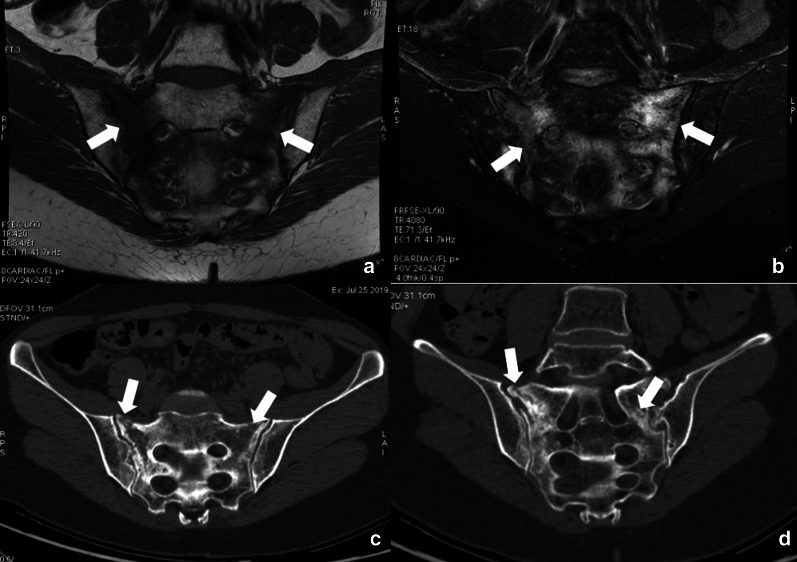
A 54-year-old woman, who underwent RT/ChT for cervical cancer, complained of low back pain 8 months after treatment completion. MRI exam with coronal oblique T1-weighted and fat suppressed T2-weighted images showed extensive bone marrow edema of the sacrum (arrows in a, b) due to insufficiency fractures clearly identified also by CT images (arrows in c, d)
